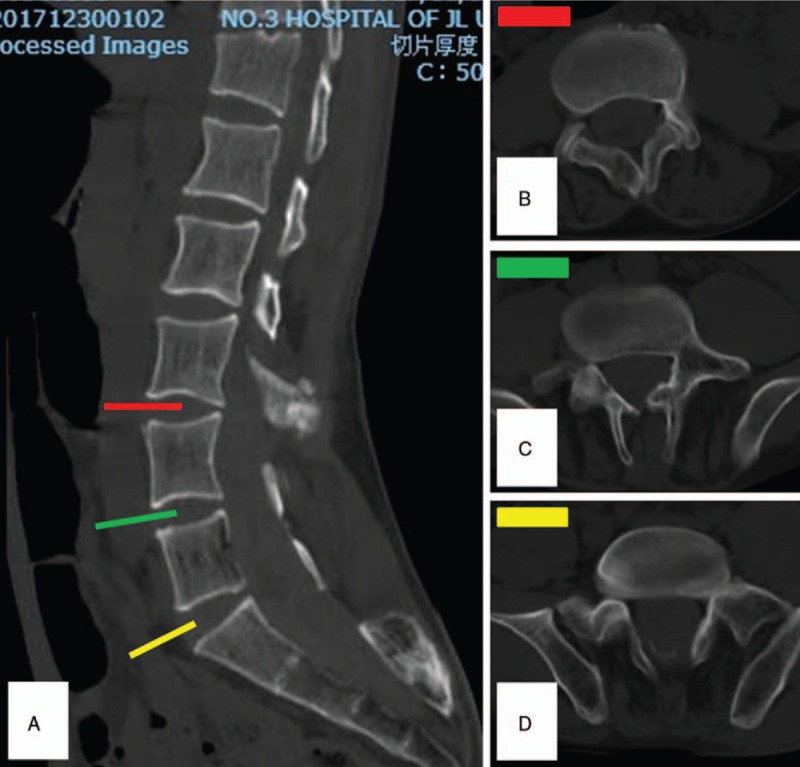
(A) The mid-sagittal reconstructed computed tomography (CT) showed the abnormal morphology of the vertebral bodies and spinous processes. (B–D) Axial CT demonstrated the vertebral laminae from L3 to L5 were more or less absent.
